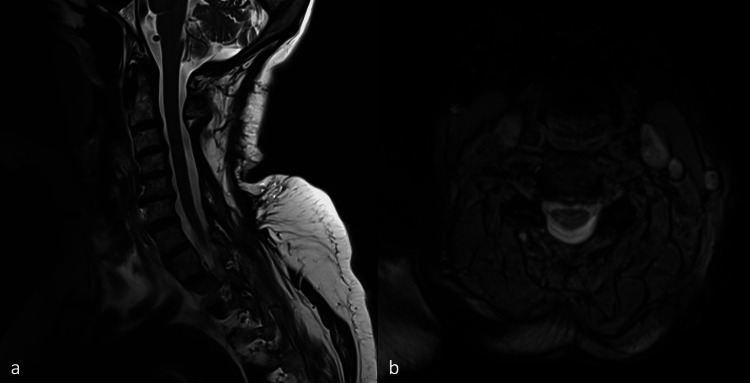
T2-weighted MRI of the cervical spine six months postoperatively. (A) Sagittal view. (B) Axial view at the C3-4 level.
